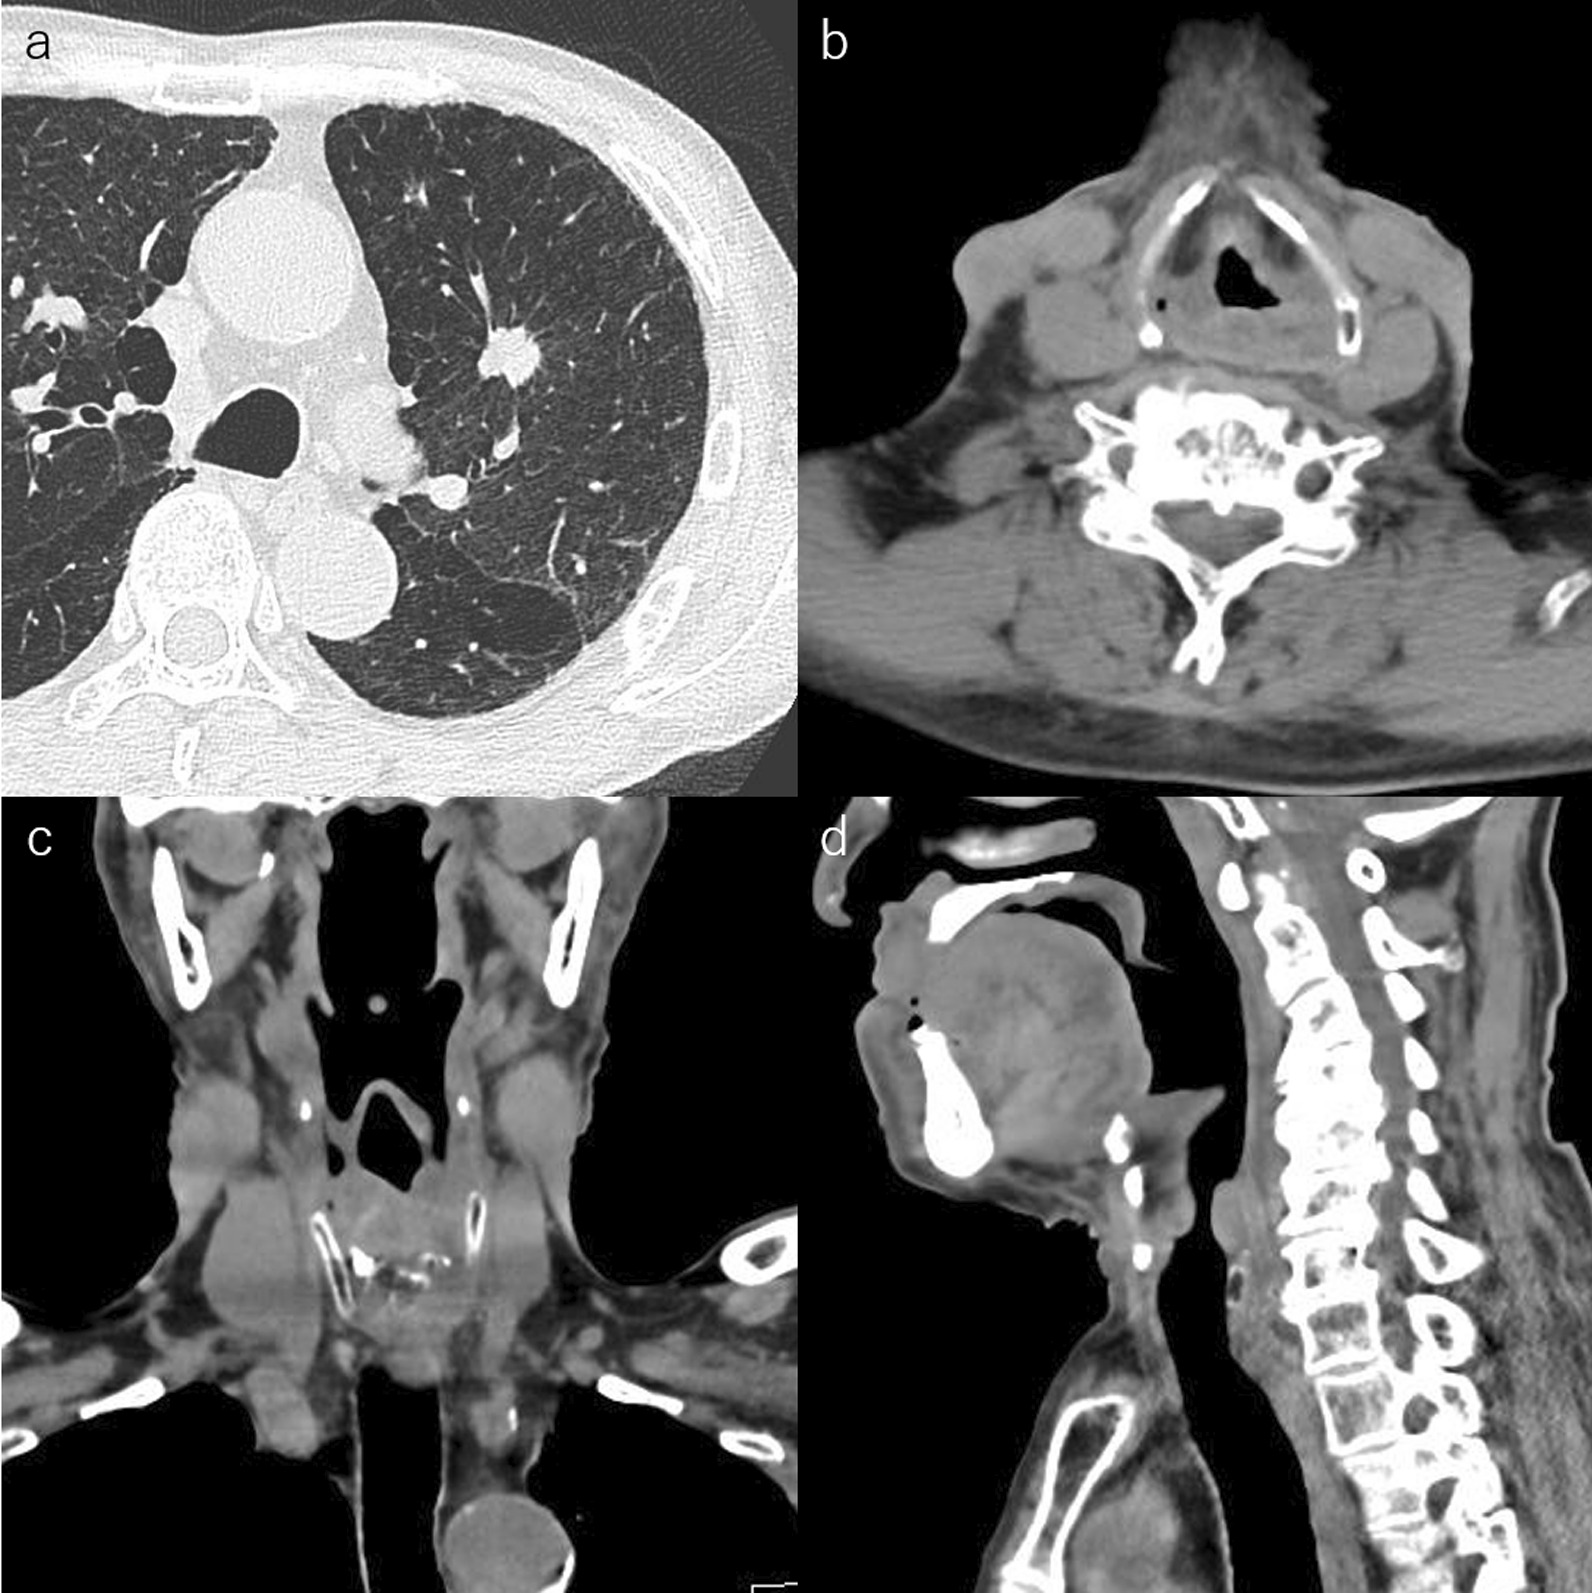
Computed tomography images. Computed tomography showing a a solid nodule shadow in left ventral segment (S3), and laryngeal edema. b Horizontal view, c coronal view, and d Sagittal view
